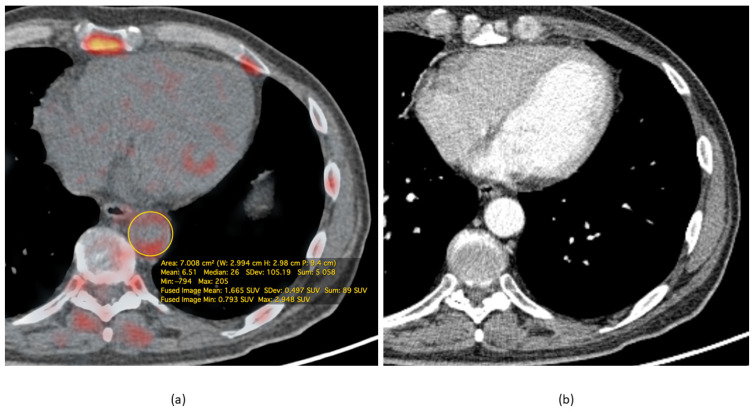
Representative FDG PET/CT of a male 65-year-old patient, diagnosed with small cell lung cancer. The study was performed 60 min after intravenous injection of 185 MBq of FDG. (a) Fusion image demonstrates increased FDG uptake in the thoracic descending aorta walls with the maximum standardized uptake value(SUVmax) = 2.9. (b) The arterial phase of the contrast-enhanced CT at the corresponding level shows only mild thickening of the vessel wall. These findings suggest an active inflammation process in the vessel walls. All calculations were performed with OsiriX MD (Pixmeo SARL, Bernex, Switzerland).
